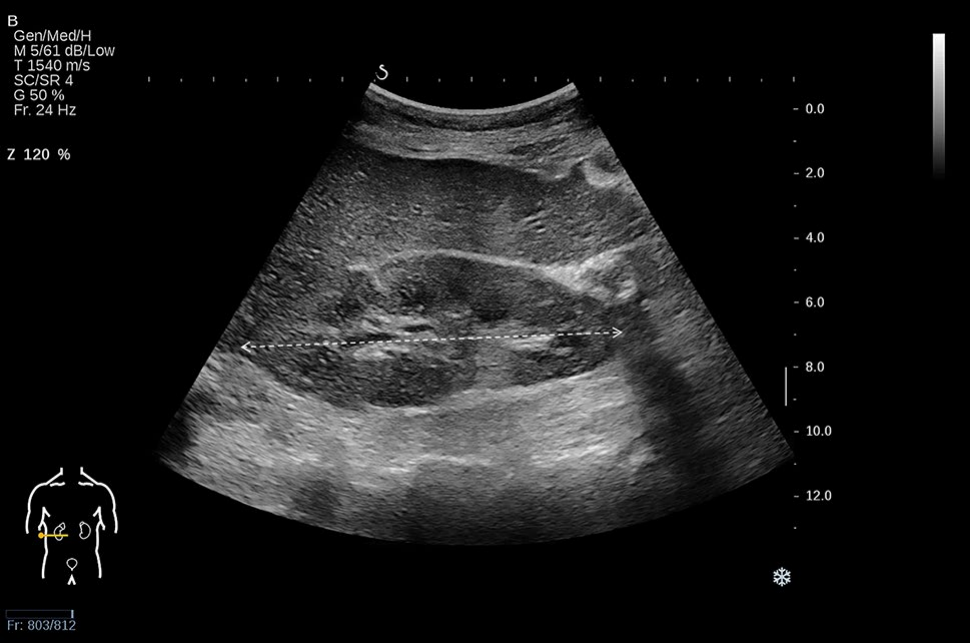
Longitudinal sonogram of the right kidney in a non-diabetic patient with chronic kidney disease. The dotted line indicates the maximal kidney length
